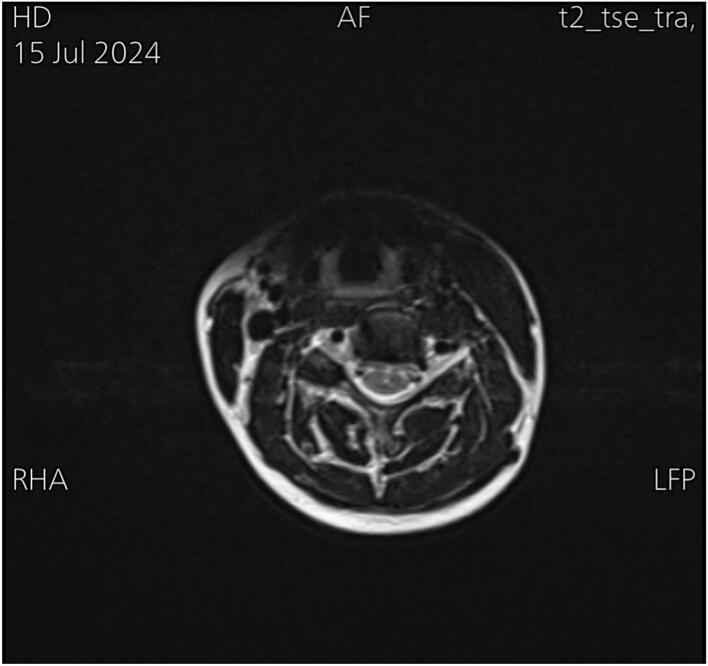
Axial T2-weighted MRI scan of the neck showing relative atrophy of the right sided sternocleidomastoid muscle with comparison to the left.
